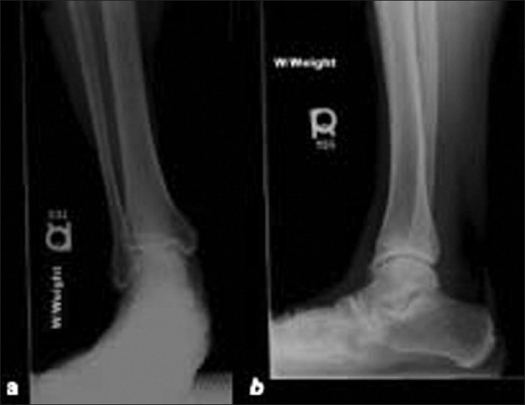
(a) Weight-bearing anteroposterior and (b) lateral radiograph of the right ankle demonstrating an anterior ankle osteophyte with medial talar dome sclerosis, suggestive of a medial talar dome osteochondral defect
